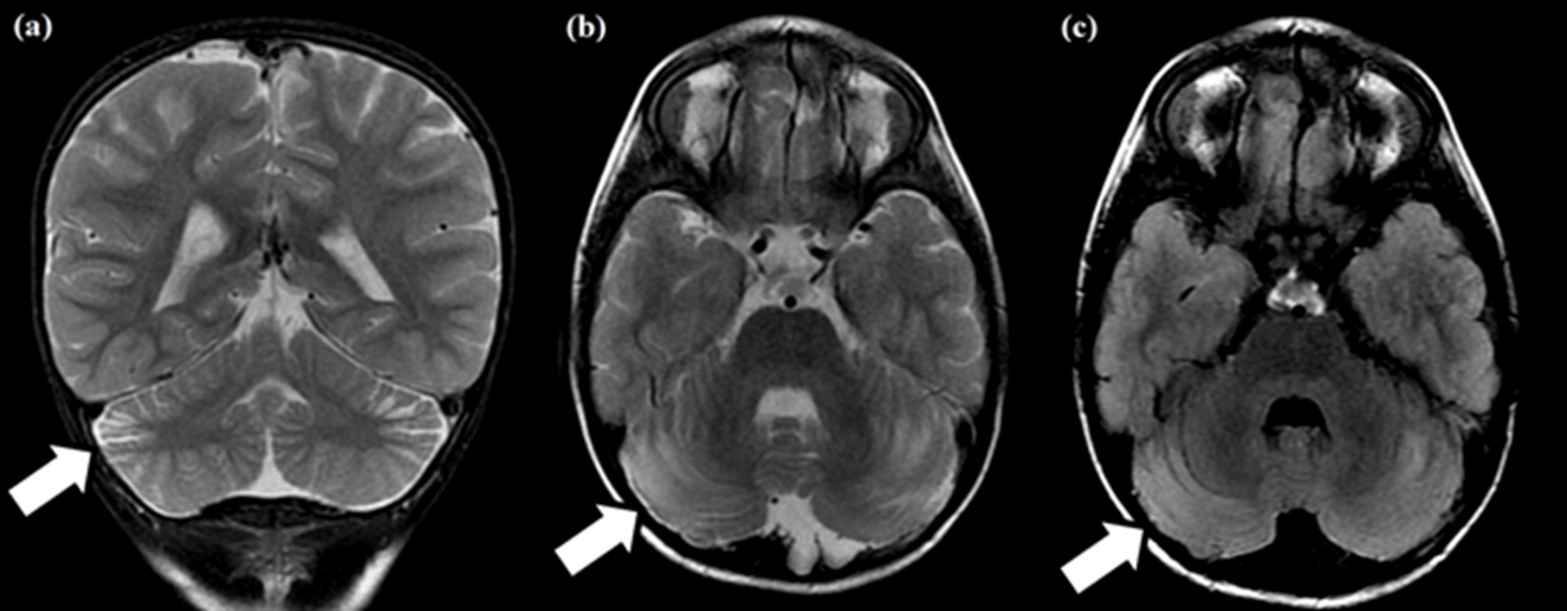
Coronal T2-weighted (a), axial T2-weighted (b), and fluid-attenuated inversion recovery (c) MRIs. Cortico-subcortical bands with hyperintensities are noted in the periphery of the middle third of the cerebellar hemispheres, associated with slight regional volumetric reduction (white arrows). These findings likely indicate regional gliosis with slight atrophy.
